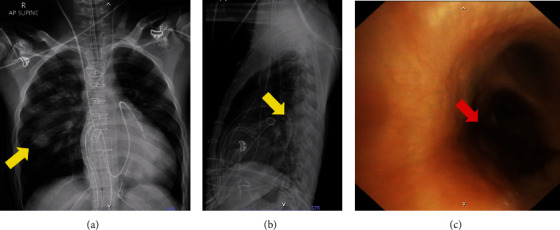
Plain AP and lateral chest X-ray and bronchoscopic finding. (a, b) Comparison of chest X-ray views indicating a suspected superior segment of right lower lobe lesion (yellow arrows). (c) Bronchoscopic findings reveal blood streak on bronchus intermedius (red arrow).
